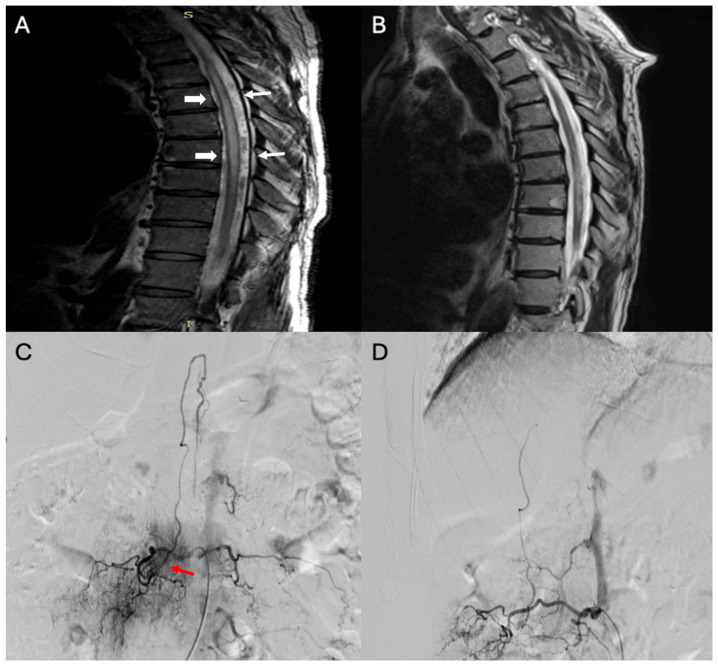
Representative imaging findings in a patient with spinal dural arteriovenous fistula (sDAVF). (A) Pre-treatment sagittal T2-weighted MRI showing tortuous and dilatated perimedullary blood vessels (small white arrows) and myelopathy (white arrows). (B) Follow-up sagittal T2-weighted MRI showing myelopathy in regression without dilatated perimedullary blood vessels in comparison with preprocedural MRI. (C) Pre-treatment digital subtraction angiography (DSA) with fistula point (small red arrow). (D) Post-treatment DSA confirming complete occlusion.
